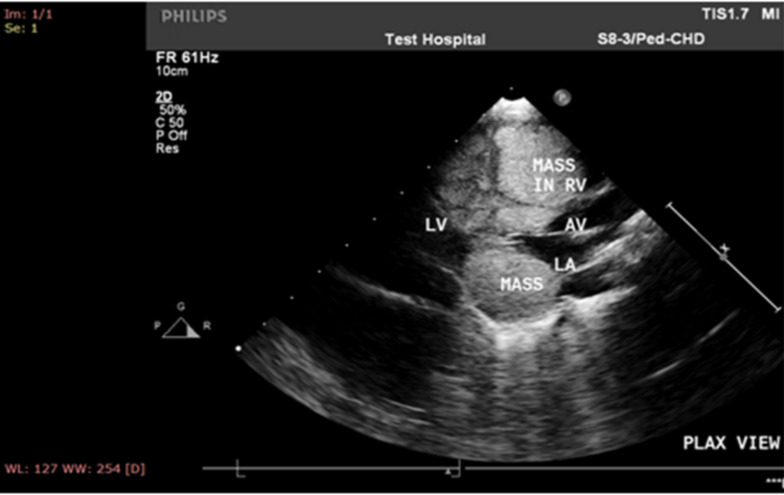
2D-echocardiographic image showing large echogenic mass with in the left atrium, the IVS and right ventricle
